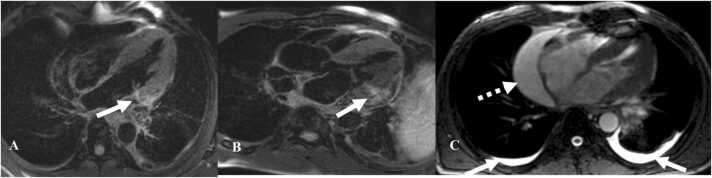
Immune-mediated myocarditis in a 67-year-old man with non-small cell lung cancer treated with Durvalumab. A&B. Post contrast axial MRI images show enhancement of the lateral and basal part of the left ventricle (white arrows) C. T2WI shows pericardial effusion along the right heart border (dashed white arrow) and small bilateral pleural effusions (white arrows).
